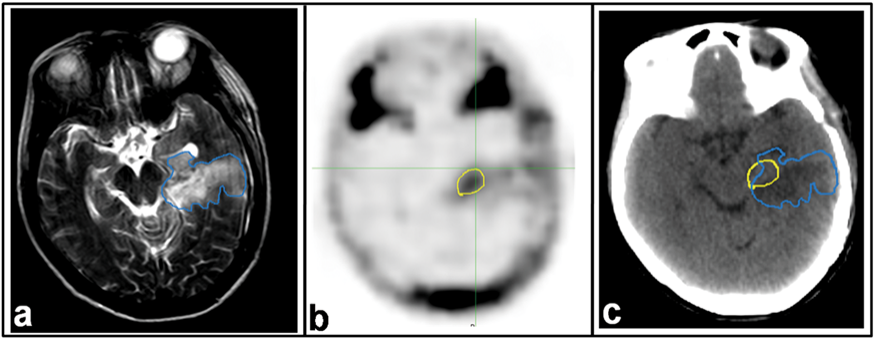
MRI and ^18^F-FLT PET-CT images from a patient with GBM. Images were taken 21 days post-operatively and 2 days before radiotherapy. a. T2-weighted MRI. b. ^18^F-FLT PET image. c. CT image of PET-CT scan. Residual tumor regions defined by T2-MRI (blue line) and ^18^F-FLT PET (yellow line) are superimposed on the CT image.
